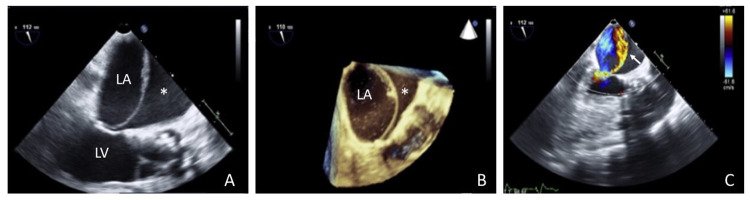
(A,B) Bidimensional and 3D visualization of left atrial dissection at transoesophageal echocardiography, with systolic expansion of the false lumen (asterisk). (C) Color Doppler showing a significant retrograde mitral regurgitation jet into the dissection chamber (white arrow) (adapted from Sardar et al. (31). LA, left atrium; LV, left ventricle.
